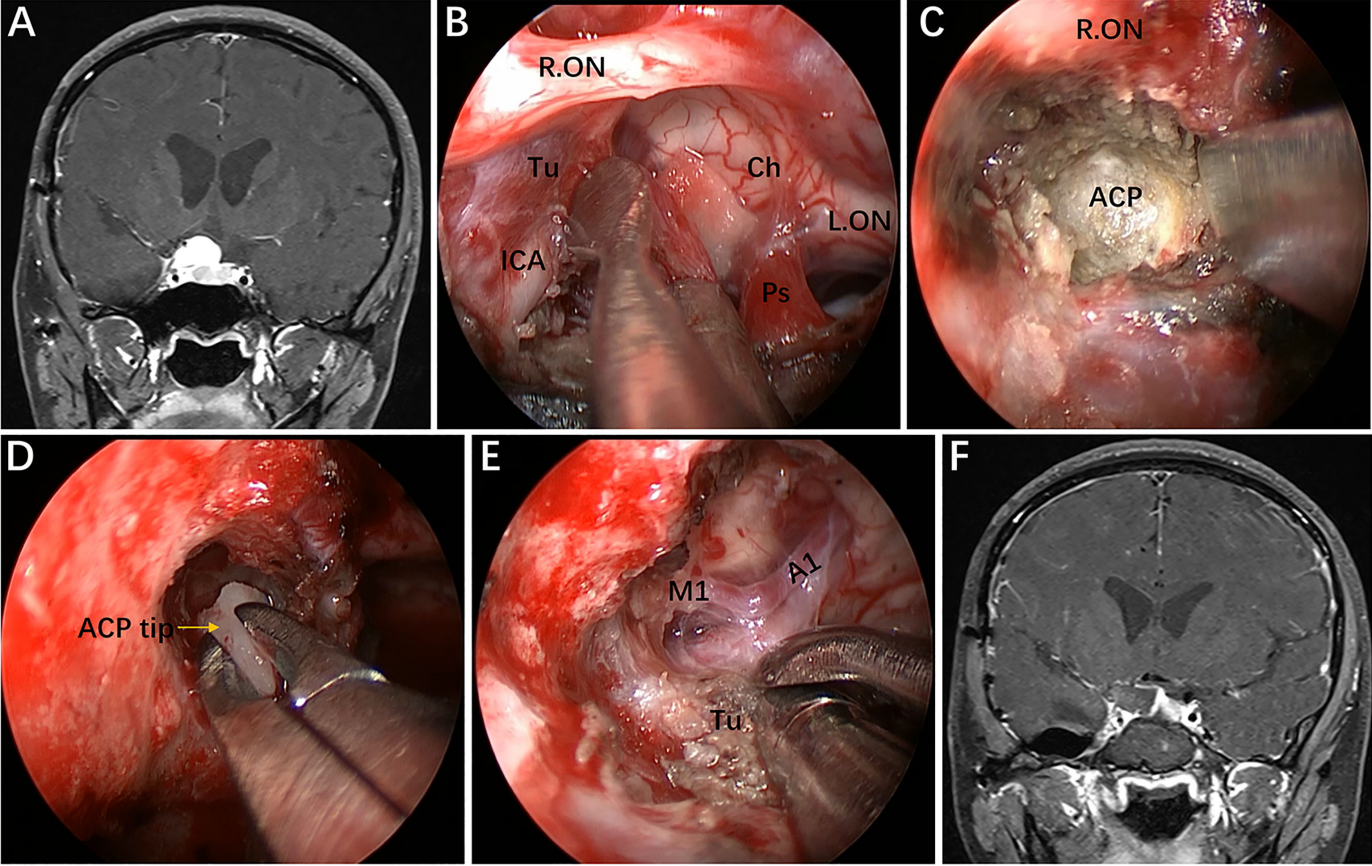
Case 2, A 25-year-old woman presented with headache and decreased vision in her right eye for 6 months. (A) Preoperative coronal Gd-enhanced MRI demonstrating a right recurrent ACP meningioma. (B) Removal of the tumor on the medial and upper parts of the supraclinoidal ICA. (C, D) Removal of the ACP invaded by the tumor. (E) Separation of tumor adhering to the bifurcation of the ICA through the infraoptic corridor. (F) Postoperative MRI showing total tumor removal. Tu = tumor; Ps = pituitary stalk; ACP = anterior clinoid process. Some panels (7A, D, F) of the figure have been published in *Journal of Neurosurge*ry. Published with permission. The figure is available in color only online.
